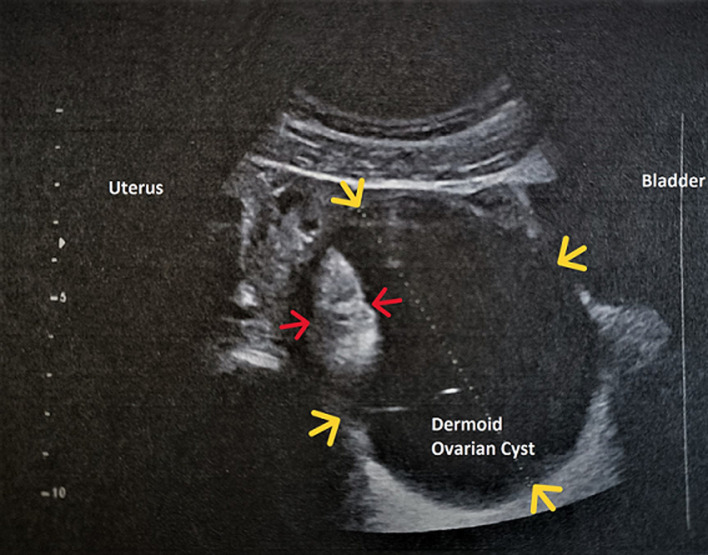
transabdominal ultrasound imaging of a twisted dermoid ovarian cyst (yellow arrows) with the typical presence of fat within it (red arrows)
